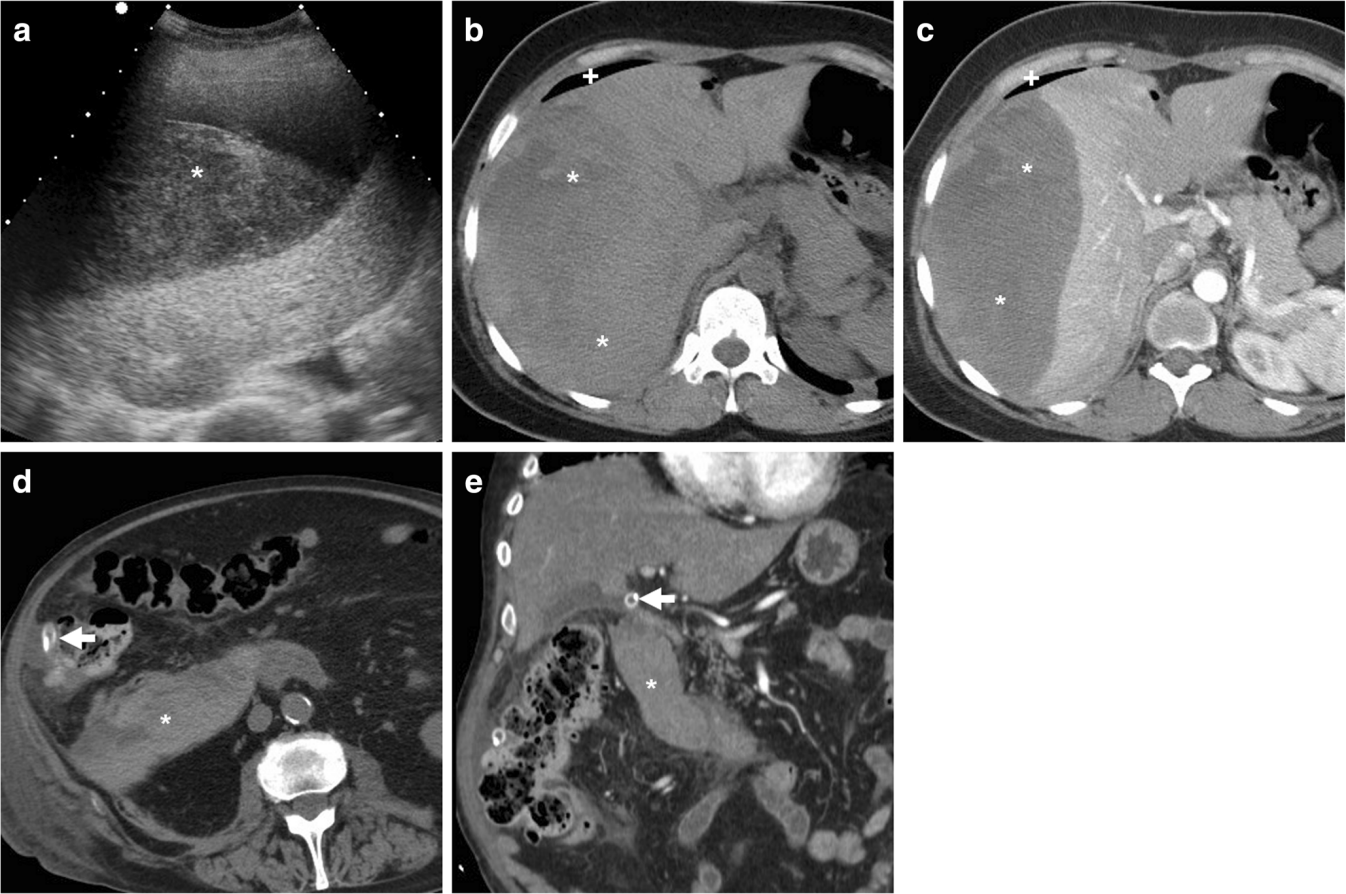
Atypical sites of post-cholecystectomy bleeding. Vast subcapsular haematoma (*) seen on ultrasound (a), precontrast (b) and enhanced (c) CT 24 h after laparoscopic cholecystectomy, causing compression of the liver, treated by percutaneous drainage and transfusions. Note residual intraperitoneal air (+) d, e Paraduodenal and anterior pararenal haematoma (*) seen on unenhanced (d) CT on the 3rd postoperative day after laparoscopic cholecystectomy, with drainage (*thick arrows*) still in place, without contrast blushes, suggesting active bleeding on arterial-phase CT (e); size and attenuation of the hematoma tended to regress at follow-up CT (not shown) on conservative treatment, including transfusions
